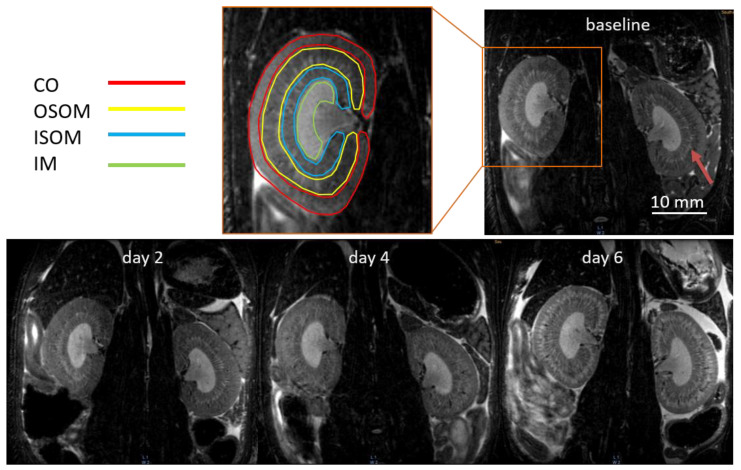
Examples of isotropic T2-weighted images of AAI-treated kidneys. At baseline, four kidney layers are outlined with contours of different colors in the zoomed image. CO, cortex; OSOM, outer stripe of outer medulla; ISOM, inner stripe of outer medulla; IM, inner medulla.
